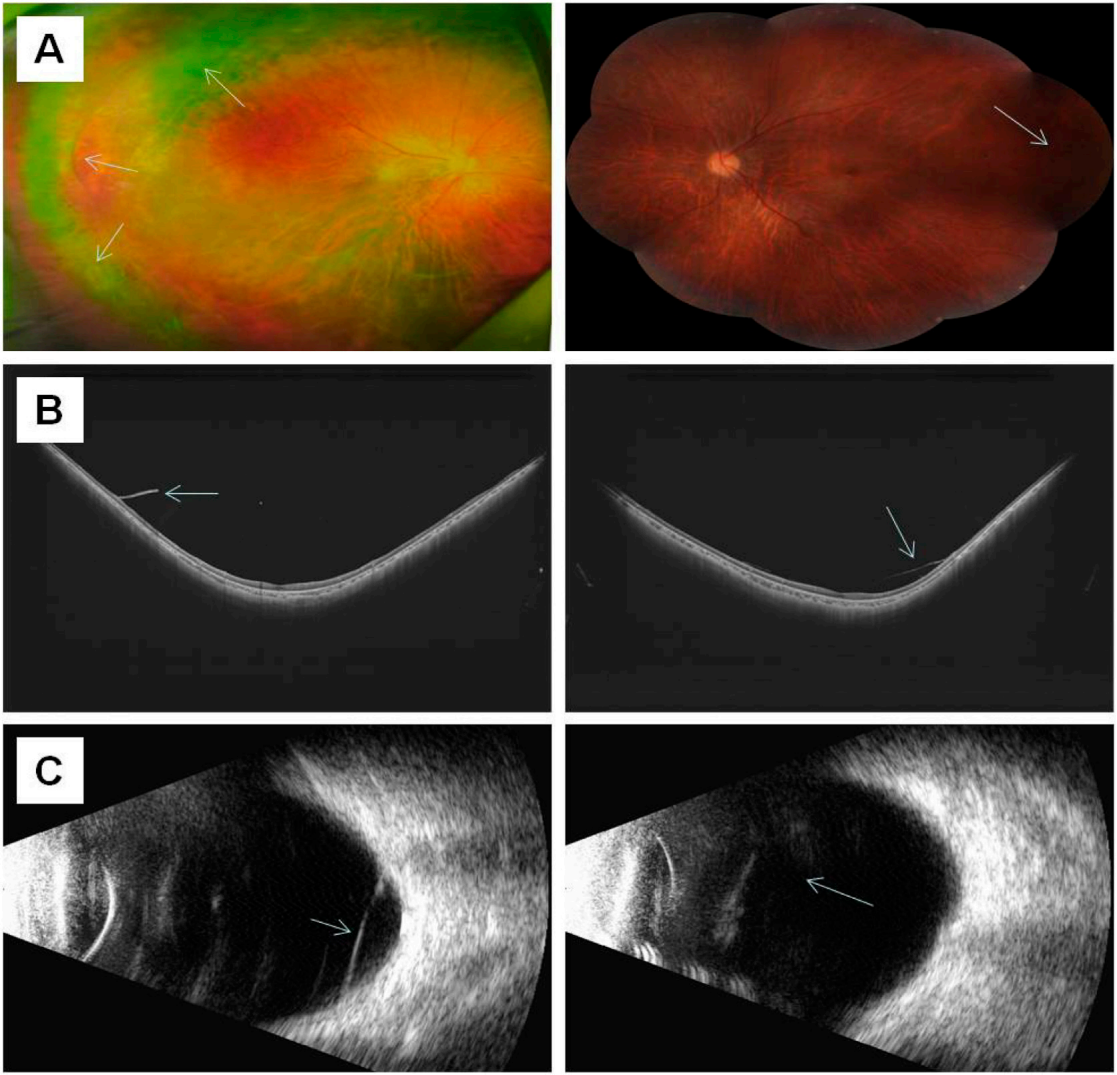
The results of ocular imaging examination of individual II-1 (A) The left and right sides represent the fundus images of the right eye and left eye, respectively; (B) The left and right sides indicate the OCT of the right eye and left eye, respectively; (C) The left and right sides indicate the ocular B-ultrasound examination of the right eye and left eye, respectively. The arrow indicates the lesion.
